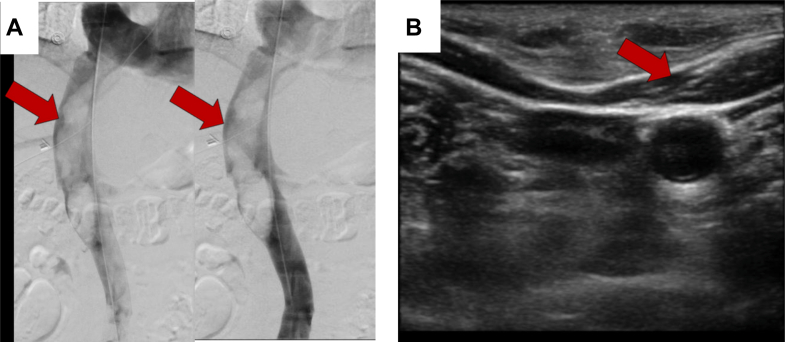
Venography and Intravascular Ultrasound Findings (A) Venography shows a large expansile intraluminal filling defect within the IVC (red arrow), extending from just below the renal veins to the cavoatrial junction. (B) IVUS demonstrates multiple intraluminal vascular channels without endothelial invasion or adherence, indicating a vascular intraluminal tumor rather than thrombus. These findings support the diagnosis of intravenous leiomyomatosis. Additional dynamic imaging is provided in Videos 5 and 6. IVC = inferior vena cava; IVUS = intravascular ultrasound.
